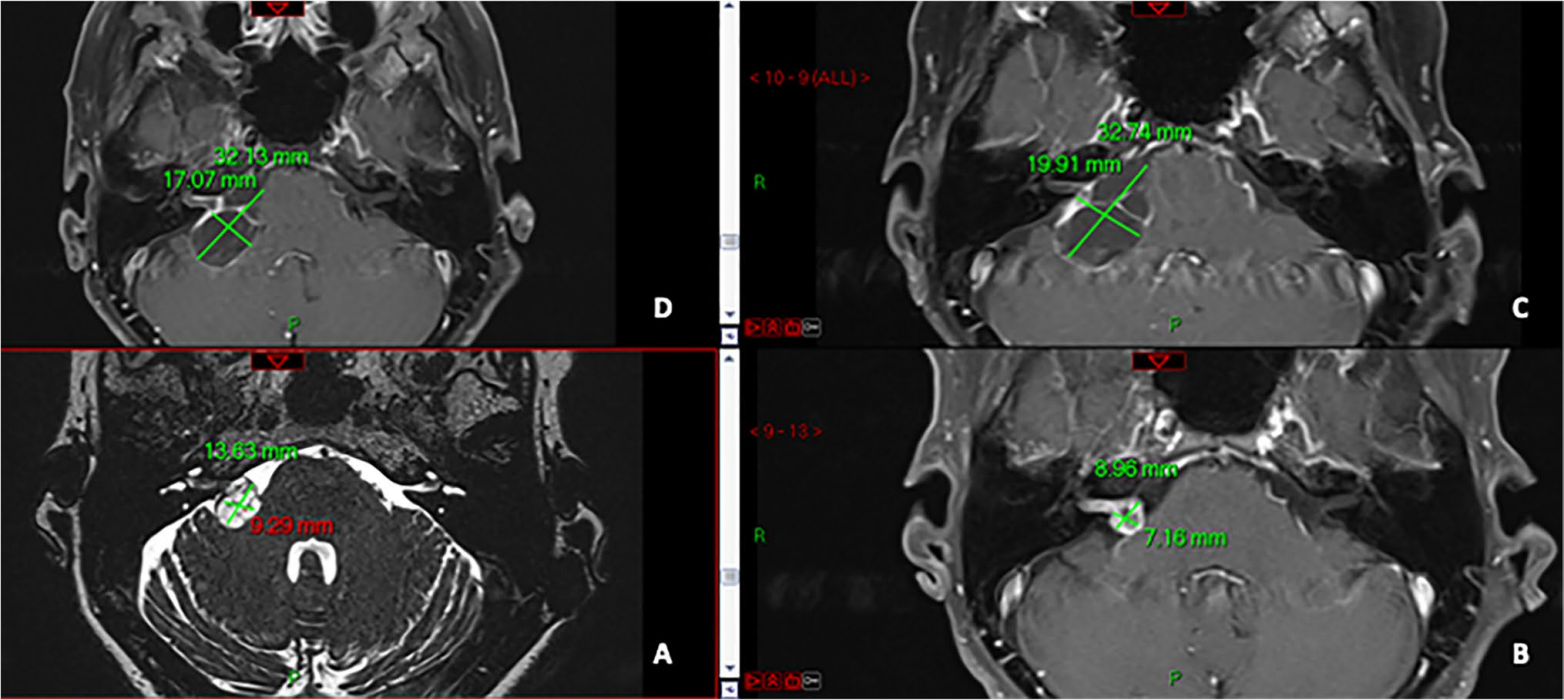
The changes in the size of a right VS in an elderly patient in axial 1.5 T MRI (anticlockwise): A steady state MRI prior to treatment showing the right VS with 13.6 mm maximum intracranial diameter, with good response (post-gadolinium T1-weighted) following the treatment at just under 9 mm diameter (B); cystic degeneration 30 months post-SRS with significant enlargement and temporal balance deterioration with just under 33 mm maximum intracranial diameter (C) and stable appearances (with a small decrease in size) in an 18-month follow-up following the re-growth at 32 mm (D)
